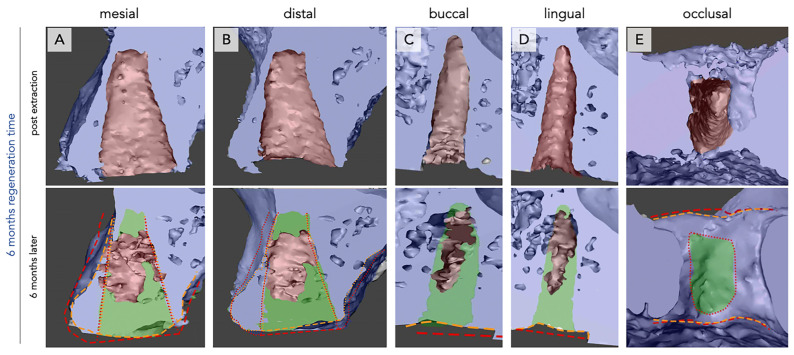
Bone regeneration and morphological changes within the premolar extraction alveolus of one rooted tooth after six months of regeneration time. Radiological imaging of the extraction alveoli using CBCT performed directly after extraction (upper row) and after six months of regeneration (lower row) in mesial (A), distal (B), buccal (C), lingual (D), and occlusal (E) orientations. New bone formation (green) and differences in alveolar crest dimensions between both time points (red = post-extraction, orange = after six months) are seen. Bone regeneration is observed to a large extent occlusally and to lower extent apically, leaving a non-regenerated empty region in the center of the alveoli (A–D). Mild lateral or vertical bone loss is seen (A–E).
